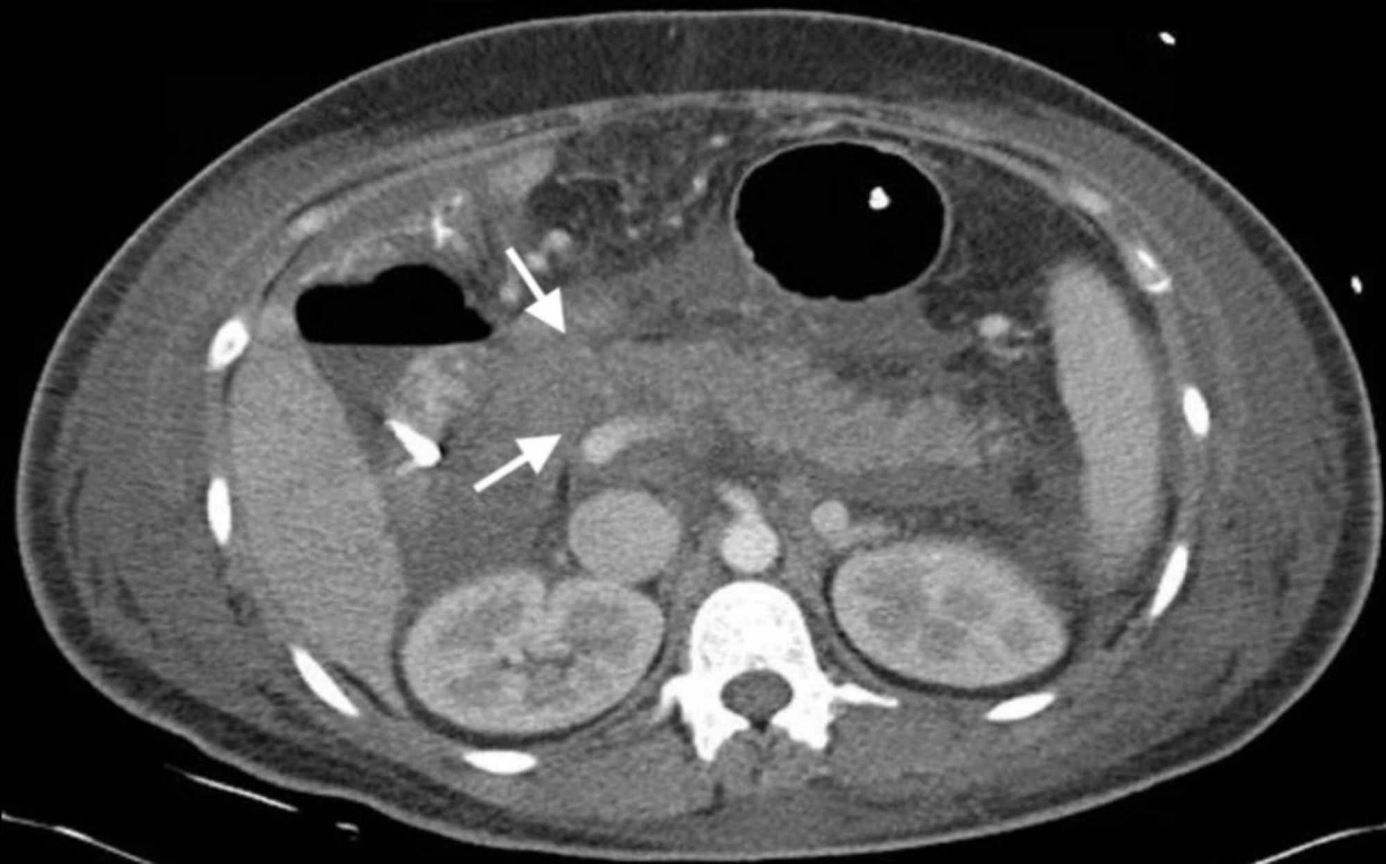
CT scan of the abdomen with post-surgical changes of pancreaticoduodenal resection (white arrows).
